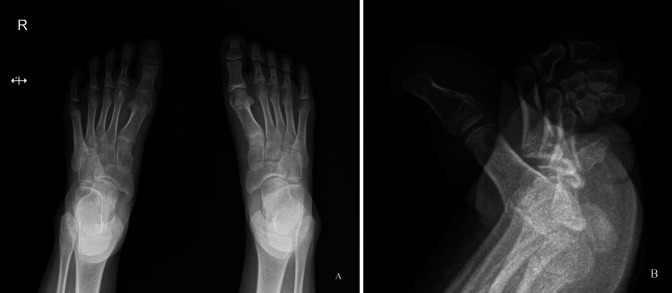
Simple anterioposterior (A) and lateral (B) radiographs of the right foot showing a 4-mm, round, radiolucent lesion with central mineralization and rim sclerosis at the head of the proximal phalanx in the great toe.
